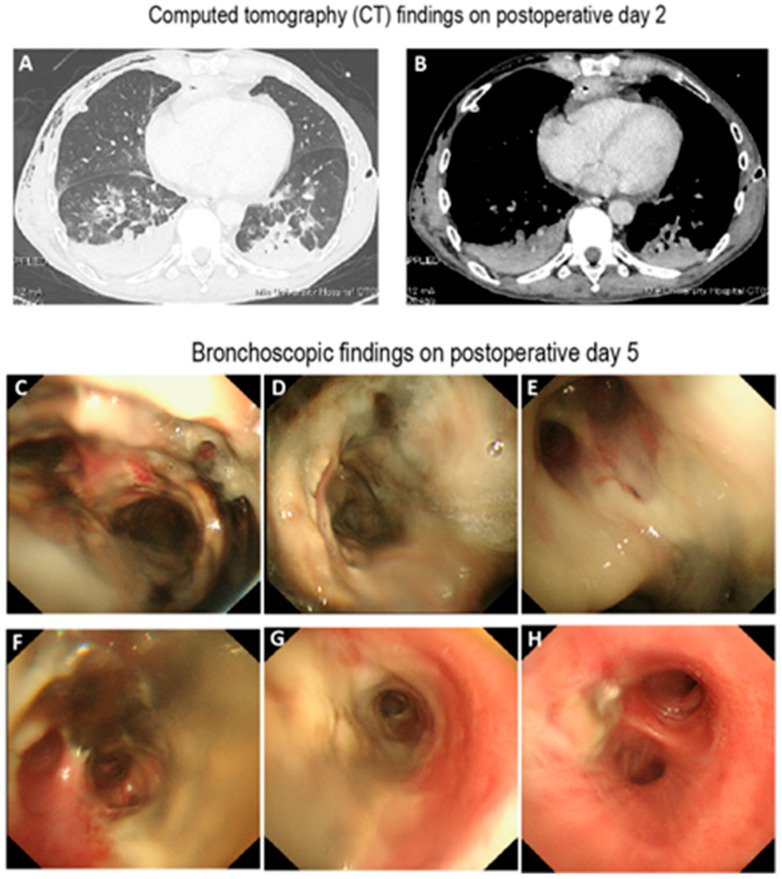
Postoperative computed tomography and bronchoscopic findings. (A,B) Computed tomography image obtained on postoperative day 2, demonstrating pneumonia, atelectasis, right-sided pneumothorax, and subcutaneous emphysema. These findings necessitated the placement of a right-sided chest tube. (C–H) Bronchoscopic images on postoperative day 7 showing widespread epithelial whitening involving the trachea (C), right main bronchus (D), orifice of the right upper lobe bronchus (E), bronchus intermedius (F), right lower lobe bronchus, and the posterior wall of the left main bronchus (G). Focal black discoloration was also observed. In contrast, the epithelium distal to the left secondary carina appeared unremarkable.
